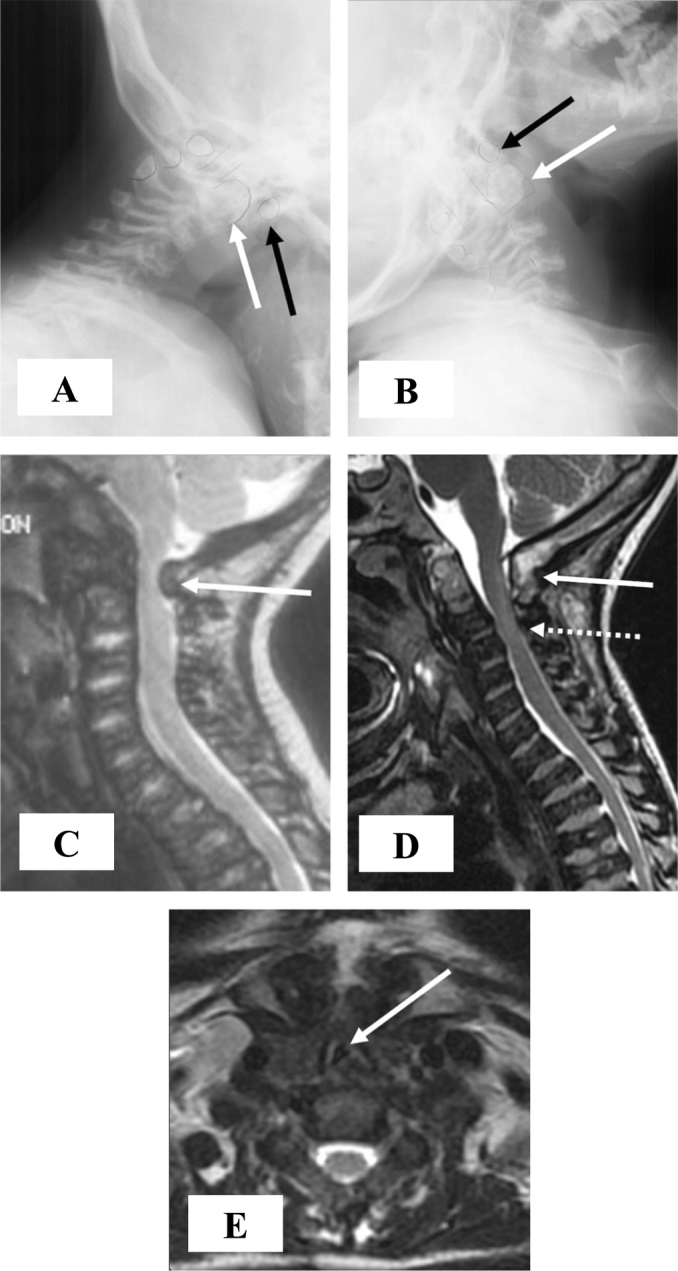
Pre- and postoperative imaging of the cervical spine. Preoperative cervical spine x-rays at age 3 years performed in flexion (A) and extension (B) show atlantoaxial instability, with bony outlines and spinal canal highlighted in pencil. The anterior arch of C1 (black arrows) rides up of the hypoplastic dens (white arrows) in extension, and moves anteriorly in flexion causing decreased space available for the spinal cord. (C) A preoperative sagittal T2-weighted MR image of the cervical spine at age 4 years shows spinal cord impingement by the posterior arch of C1 (arrow). Occipital to C2 posterior decompression and fusion was performed shortly afterwards. (D) A subsequent sagittal T2-weighted MR image of the cervical spine at age 16 years shows mature occipital to C2 bony fusion (arrow) with diffuse spinal stenosis at lower levels (dashed arrow) but no spinal cord gliosis. (E) An axial T2-weighted MR image from the same study shows marked tracheal narrowing with triangular configuration (arrow) at the thoracic inlet.
